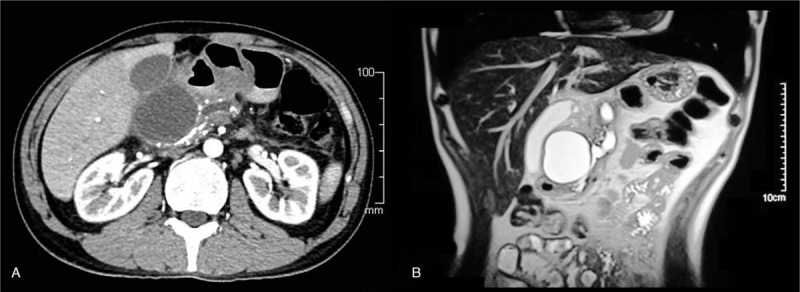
(A) The computerized tomography (CT) scan shows the upstream dilation of the pancreatic with the radiopaque stones in the pancreatic body and a large pancreatic pseudocyst (PPC) with the size of about 5.0 cm × 6.3 cm of the pancreas. (B) Magnetic resonance cholangiopancreatography reveals the upstream dilation of the pancreatic with the radiopaque stones in the pancreatic body and a large pancreatic pseudocyst (PPC) with the size of about 5.0 cm × 6.3 cm of the pancreas. PPC = pancreatic pseudocyst.
